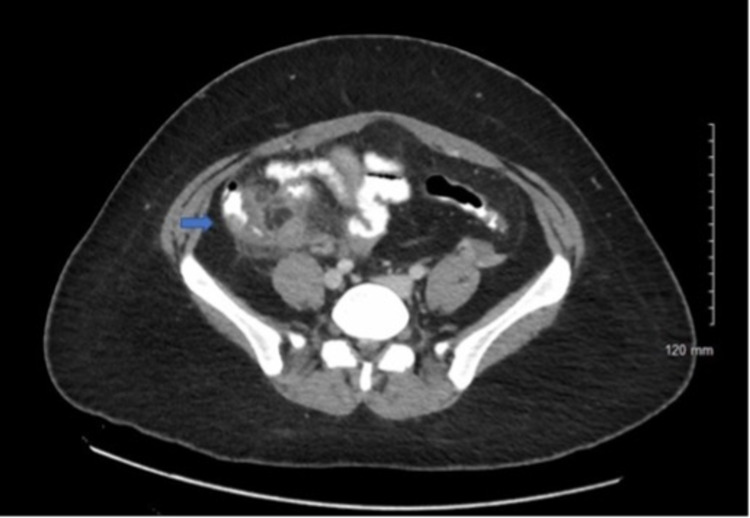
CTAP with contrast: transverse view demonstrating inflammatory thickening of cecum CTAP, Computed tomography arterial portography.
